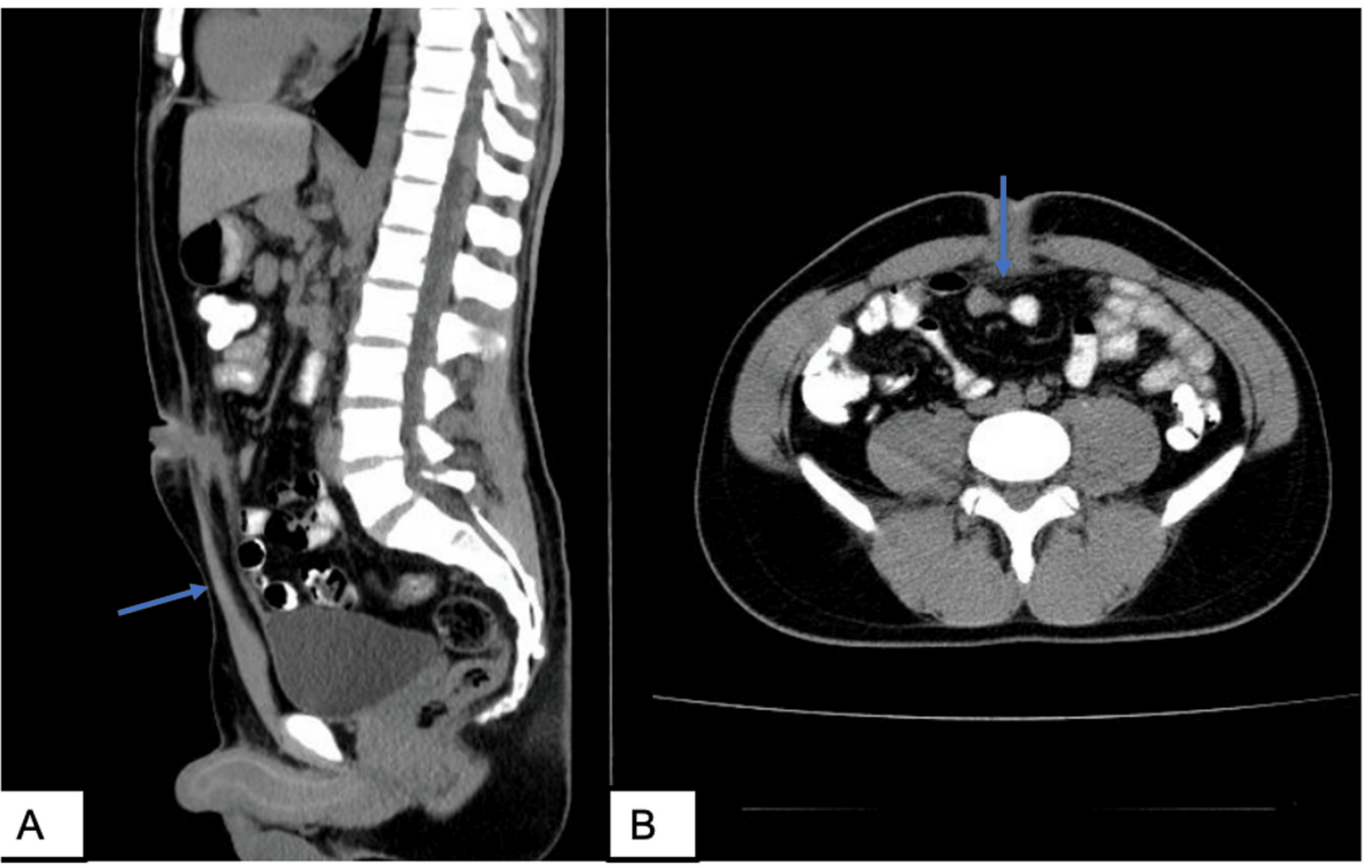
Contrast-enhanced abdominal and pelvic CT scan: A. Sagittal view: Hypodense tubular structure extending from the bladder dome to the anterior abdominal wall. B. Axial view: Hypodense structure with surrounding mesenteric fat stranding.
